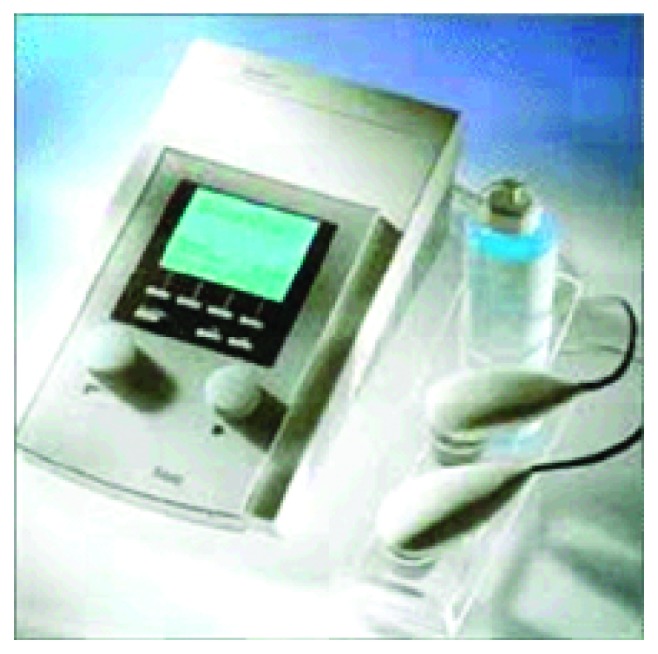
SonoStim®, a deep heat ultrasound device (Zimmer MedizinSysteme GmbH, Neu-Ulm, Germany).
